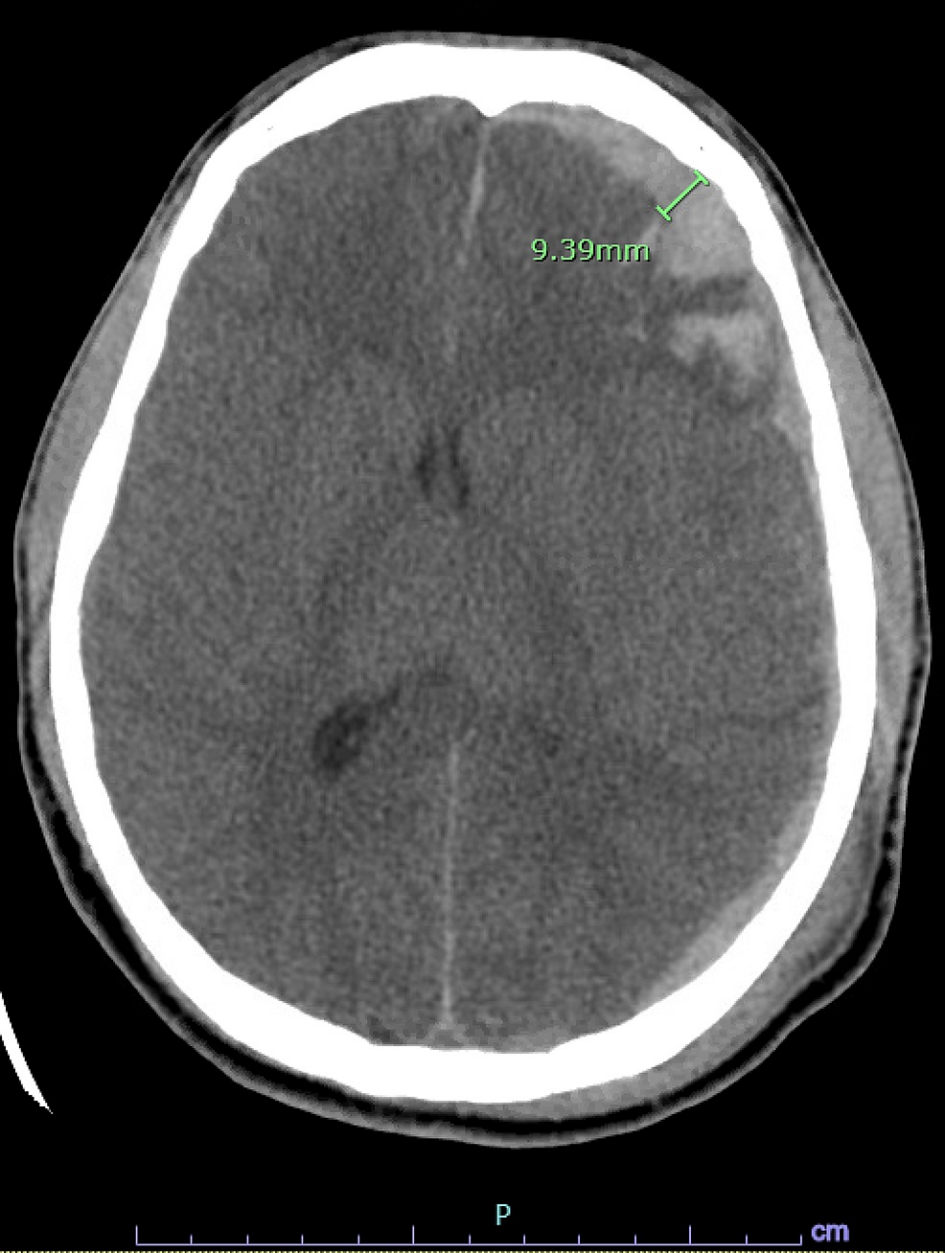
CTA head/neck. Intraparenchymal hemorrhage and 9 mm subdural hemorrhage were visualized in the left frontal lobe and left temporal lobe. CTA: computed tomography angiography.
